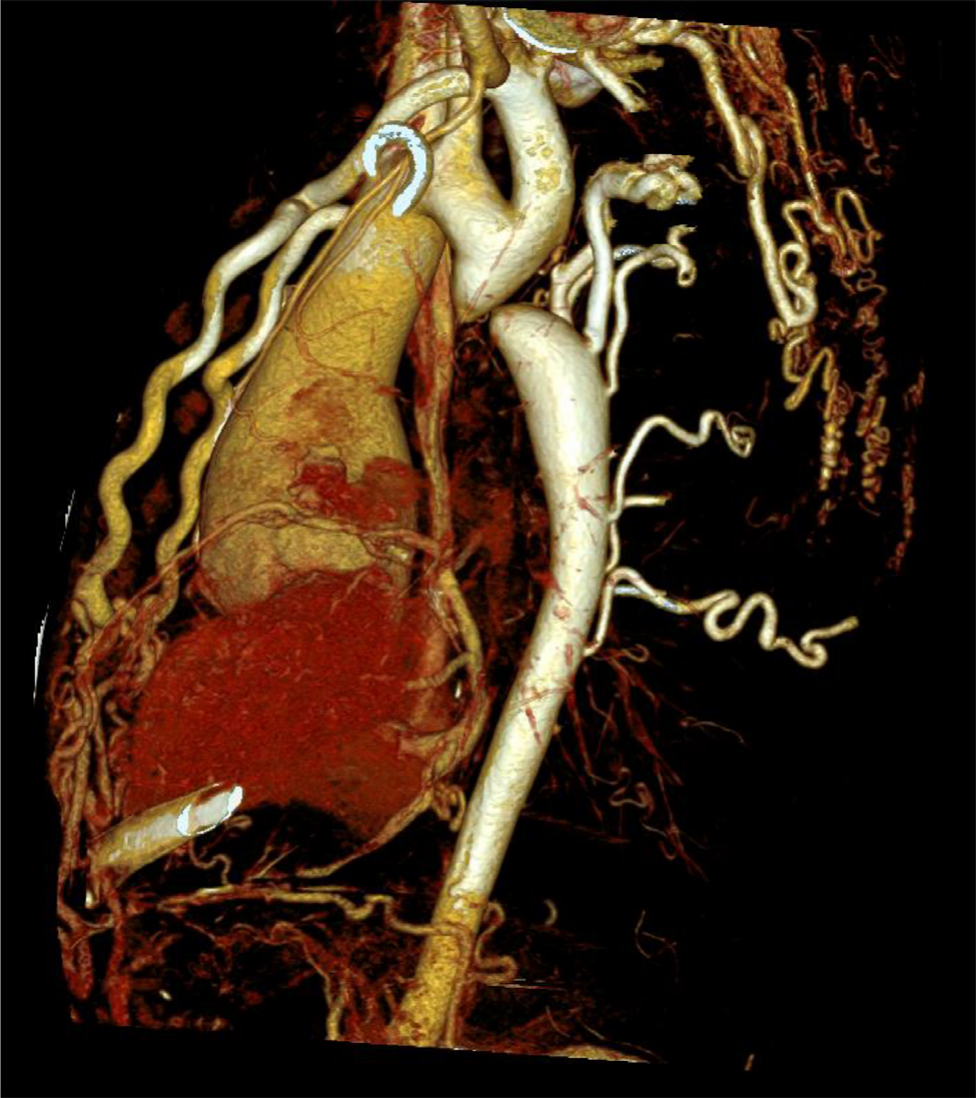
Virtual reconstruction CT scan image demonstrating the coarctation site.
